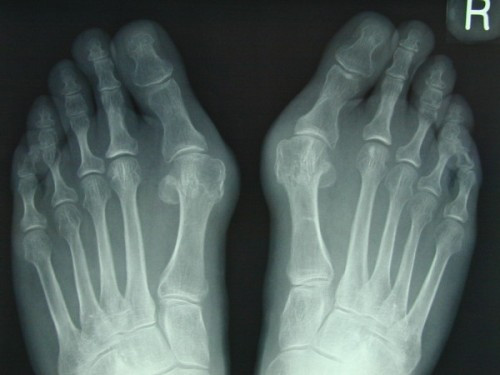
Rotation scarf and Akin osteotomies pre-operative x-ray.
